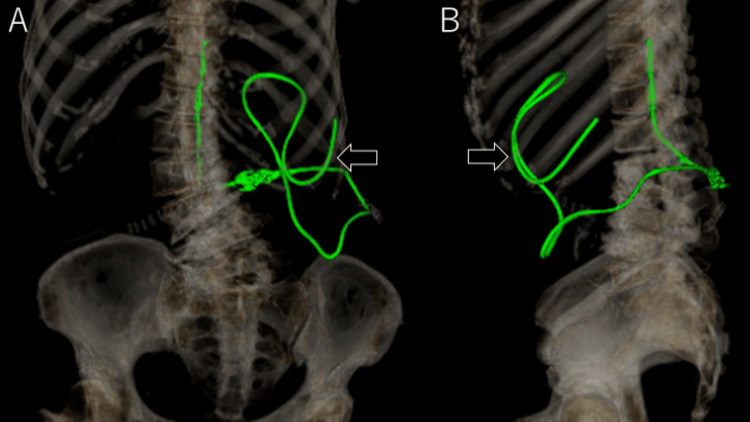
3D CT following LP shunt revision (A: frontal view, B: left lateral view) Post-revision 3D CT scans show the distal catheter inserted into the left abdominal cavity (arrows).
